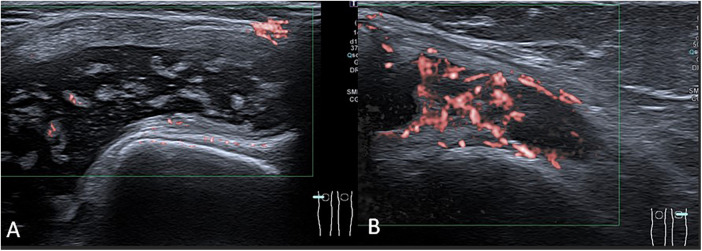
Ultrasound images of the lateral parapatellar recess of the knee in a patient with CACP (A) and a patient with JIA (B) are shown. Both images demonstrate large effusions (OMERACT BM grade 3). Panel A shows synovial hypertrophy and multiple hyperechoic foci suggestive of increased viscosity, whereas Panel B shows synovial hypertrophy without the hyperechoic foci. In addition, marked intrasynovial hypervascularity (DM grade 3) is present in Panel B but is absent in Panel A. BM, B-mode; CACP, Camptodactyly-arthropathy-coxa vara-pericarditis syndrome; CI, confidence interval; DM, Doppler mode; JIA, juvenile idiopathic arthritis.
